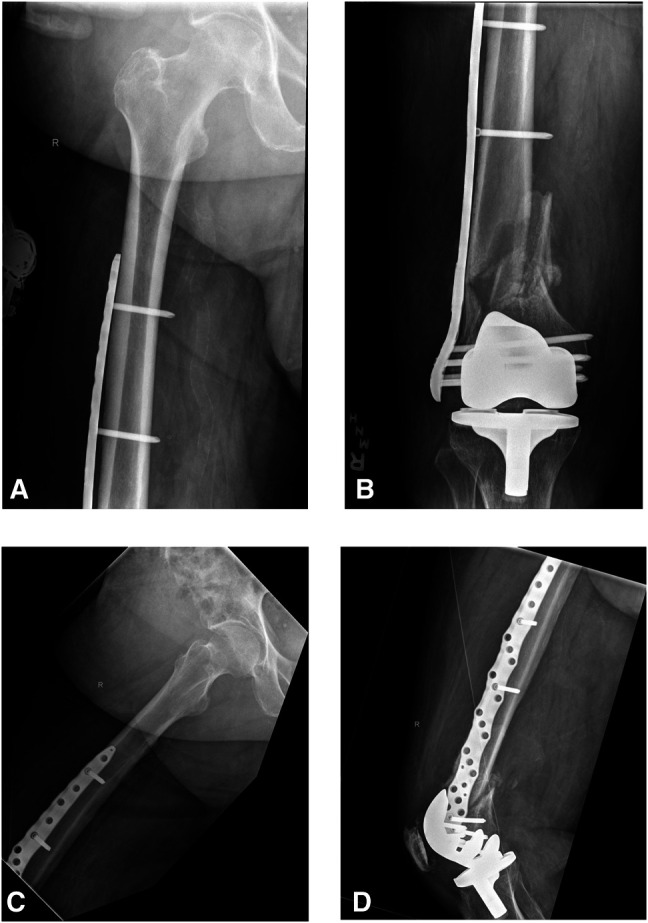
A and B, AP and (C and D) lateral radiographs of the right femur obtained 14 weeks postoperatively demonstrate slight varus malalignment of the fracture with fretting of the most distal screw proximal to the fracture line through the plate.
